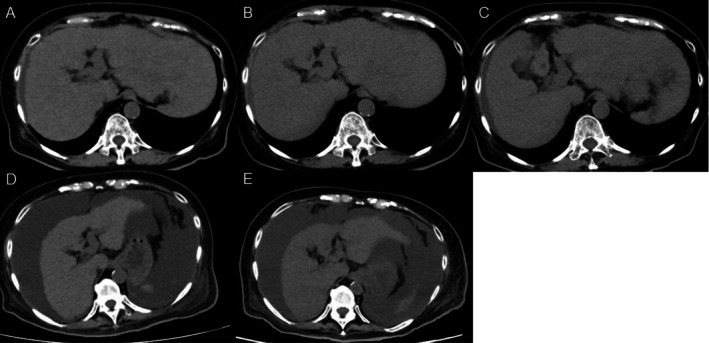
Non‐contrast axial computed tomographic images showing rapid progressive liver atrophy after durvalumab plus tremelimumab therapy. Representative non‐contrast axial computed tomographic images obtained at the portal vein bifurcation are shown at matched anatomical levels with identical window/level settings: (A) Day −1 (baseline), (B) Day 9 (admission), (C) Day 34, (D) Day 65 (marked atrophy became apparent), and (E) Day 80 (postmortem). Progressive parenchymal loss was evident over time without biliary dilatation. Day 0 indicates the initiation of durvalumab plus tremelimumab therapy on June 10, 2025.
